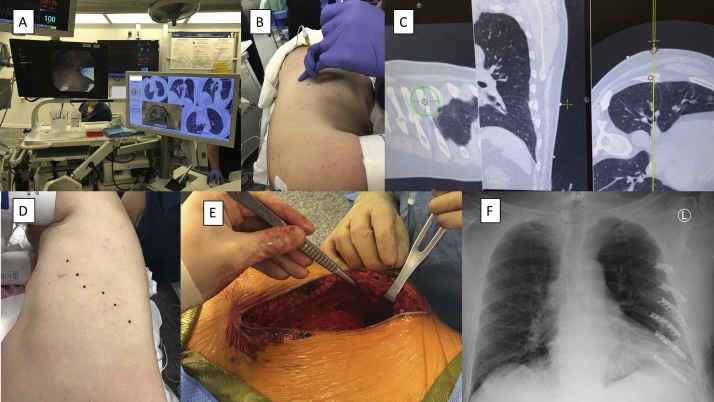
Intra-operative Localization. (A) Photograph of the bronchoscopy screen on the left and the electromagnetic navigation screen on the right correlating the bronchoscopy of carina to software-generated carina to match the computed tomographic (CT) image to the patient. (B–C) Photograph of real time localization using SPiN Perc^™^ software to mark the location of the fracture on the skin. The needle tip (B) is moved to the corresponding spot seen on the software screen (C). (D) Photograph of the skin after localization. (E) Photograph of the needle through the skin accurately locating the rib fracture. (F) Chest X-ray after rib fixation using the MatrixRIB plating system.
